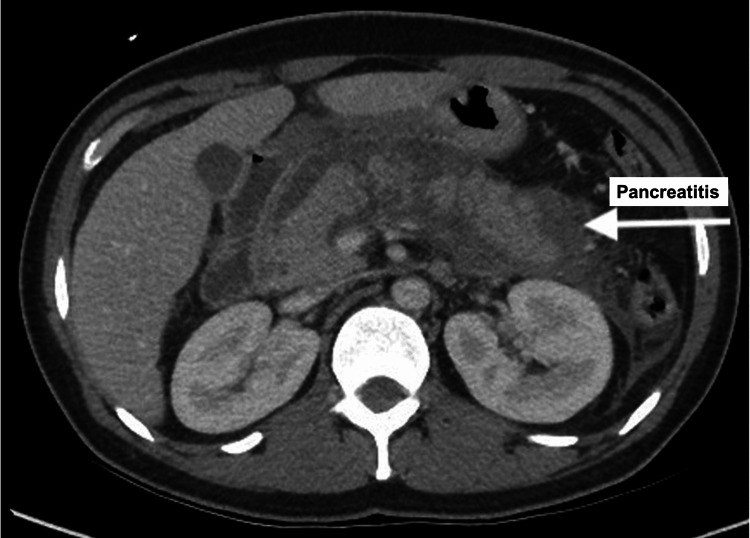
CT scan of pancreatitis without necrosis
